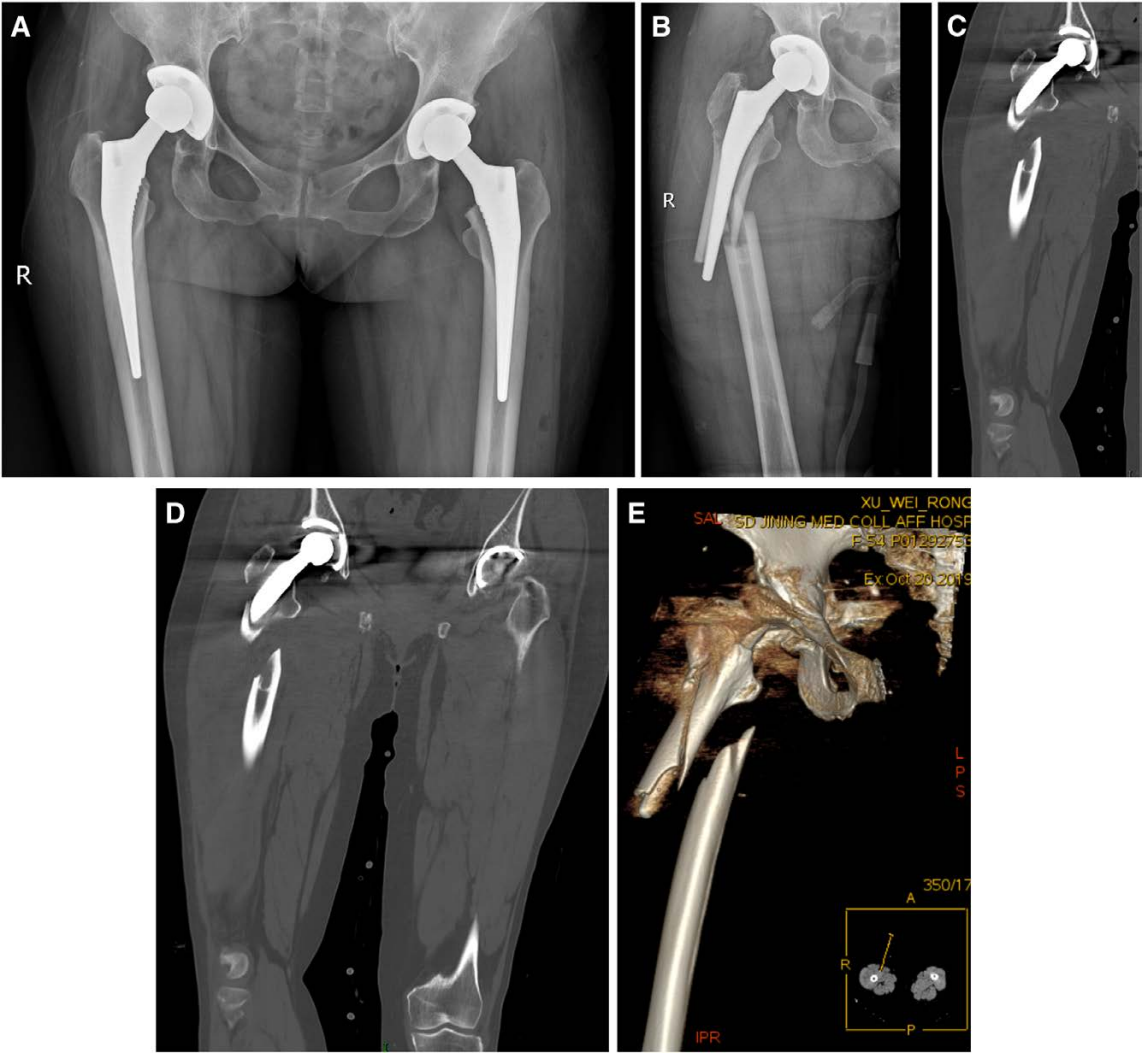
Imaging examination after bilateral THA and trauma. A: Radiography after bilateral THA; B: Radiography of the superior part of the right femur; C, D: Computed tomography (CT) scan of the right upper femur fracture after bilateral THA; E: 3 D CT reconstruction of the femoral fracture after right THA.
